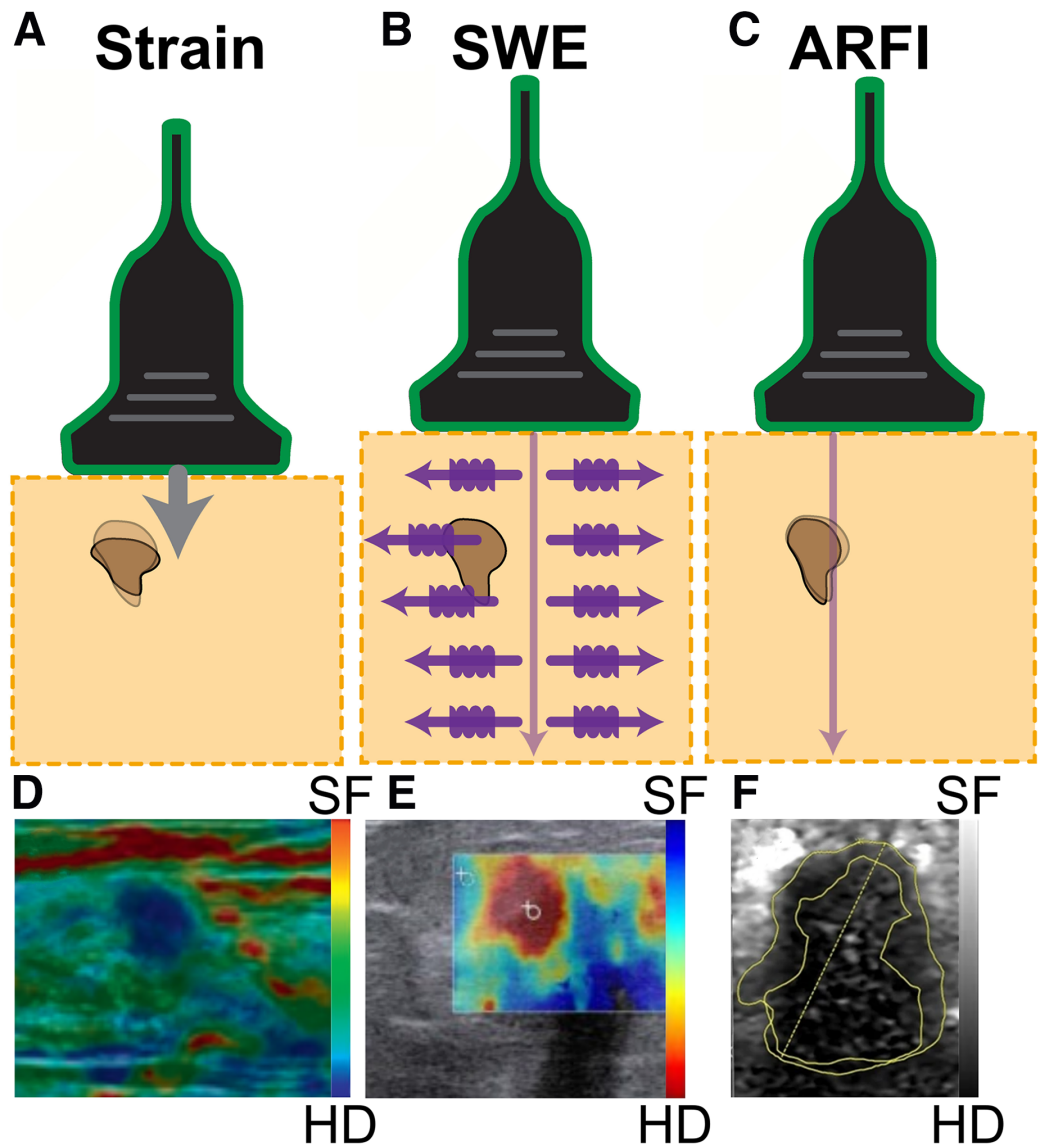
Three common ultrasound elastography modalities. (A) Strain elastography applies a physical compression force from the transducer, computing stiffness based on the resulting tissue displacements. This technique provides qualitative information on the relative stiffness between tissues, where tissues exhibiting greater resistance to the applied manual compression indicate higher stiffness. The hard nodule, indicated in dark brown, is compressed from the physical pressing of the transducer, which is represented by the arrow. The shadow of the dark mass indicates the original form of the nodule, and the fully colored form is the present form of the mass due to physical pressure being applied to it. (B) Shear wave elastography (SWE) emits a pulse wave into the tissue and tracks the perpendicularly-induced propagating shear waves. The tracked speed is computed into an elasticity value as waves travel faster through stiffer tissues including the brown lump in the figure. (C) Acoustic radiation force impulse (ARFI) imaging tracks the impulse response of the tissue after generating displacement at the region of interest with an ultrasound pulse, shown with the arrow. The change in shape of the hard nodule, indicated by the brown mass, is due to the application of ultrasound pressure. Detection of a breast mass is captured by (D) strain elastography, (E) shear wave elastography (SWE), and (F) acoustic radiation force impulse (ARFI). In each image, the breast mass was hard (HD), while the surrounding tissue was soft (SF). Images were adapted from (39, 38, 41), respectively.
